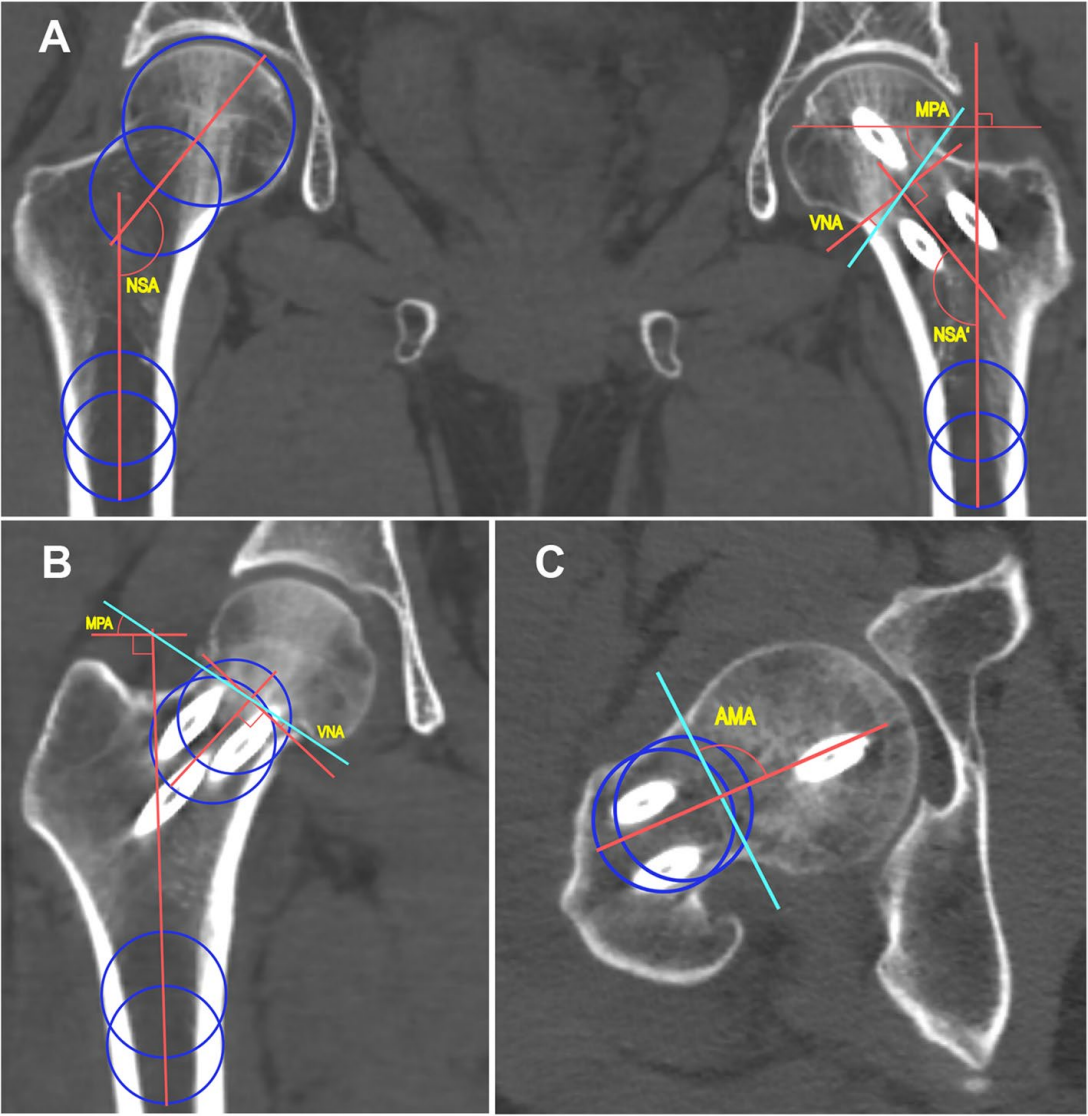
Radiographic measurements of angle parameters in coronal and axial planes. Two circles (blue) tangent to the lateral cortex of the femoral shaft and neck were drawn in each plane. The lines connecting the center points of two circles were the centerlines (red) of the femoral shaft and neck. The perpendicular lines of the centerlines were also red. The fracture lines were labeled as washed blue. A. Indirect measurement method of MPA and VNA. B. Direct measurement method of MPA and VNA. C. Measurement method of AMA
